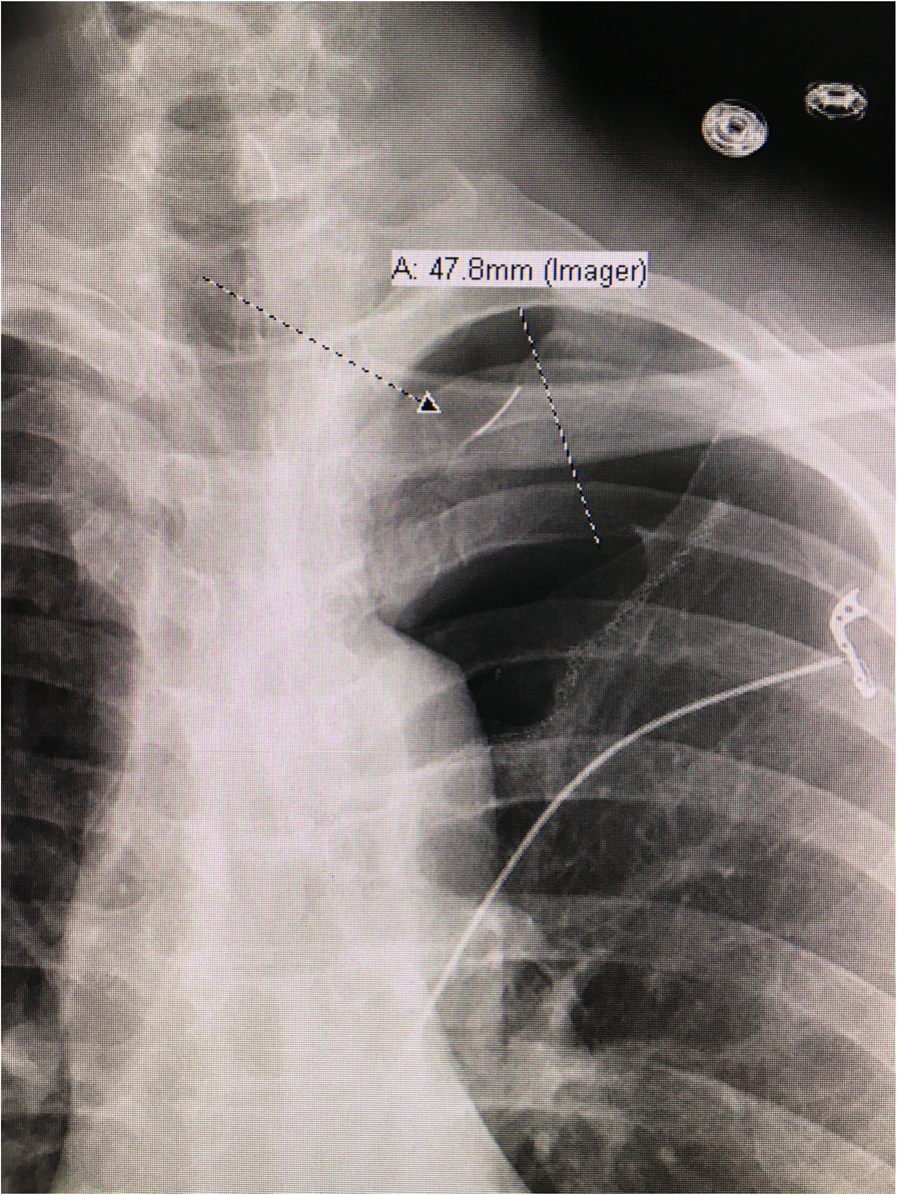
Chest x-ray after chest tube removal with apical pneumothorax and foreign body (FB)
